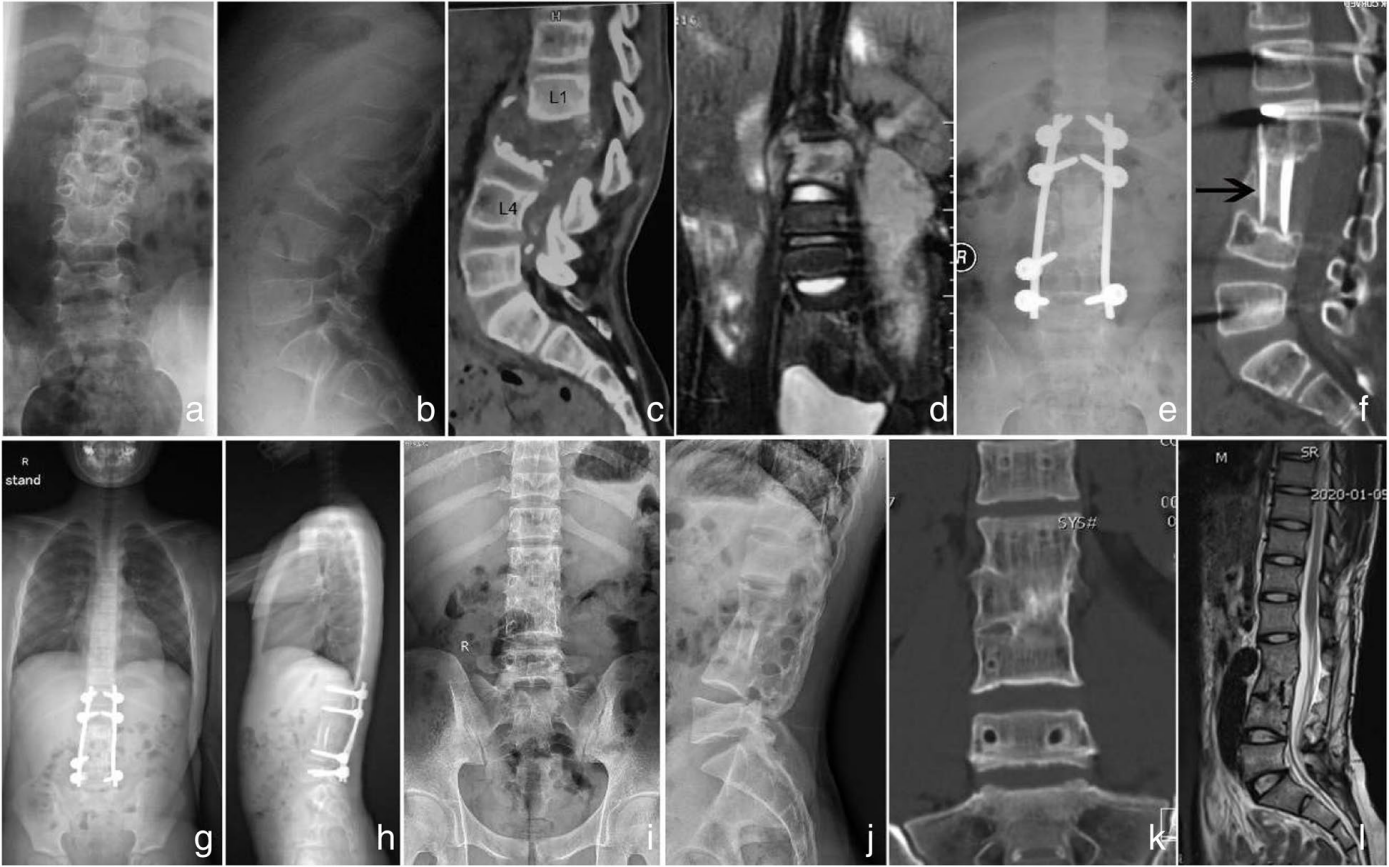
Preoperative imaging of a 7-year-old boy demonstrating L1-L3 involvement, with almost complete disappearance of L2 and L3 vertebral body resulting in spinal instability. In addition, dead bone fragments protruded into the spinal canal and there were extensive psoas abscesses (a-d). Three-month postoperative imaging showed satisfactory correction of kyphosis (e, f). Three-year postoperative X-ray showed that the position of the internal fixation and strut bone was appropriate, and the coronal and sagittal alignment was good (g, h). X-ray showed no obvious coronal or sagittal deformities 12 years postoperatively (i, j). Solid fusion was achieved (k). MRI revealed that the spinal canal was unobstructed and the spinal cord was not compressed (l)
